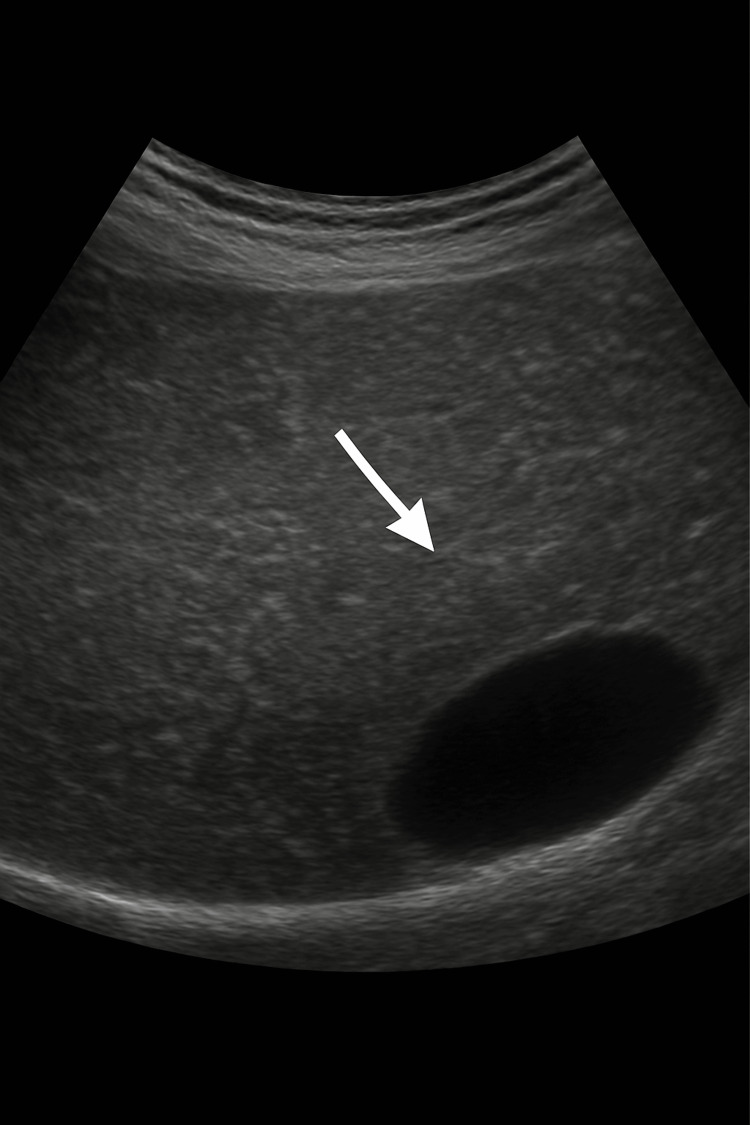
Abdominal ultrasound showing features of hepatic steatosis with increased echogenicity of the liver parenchyma
